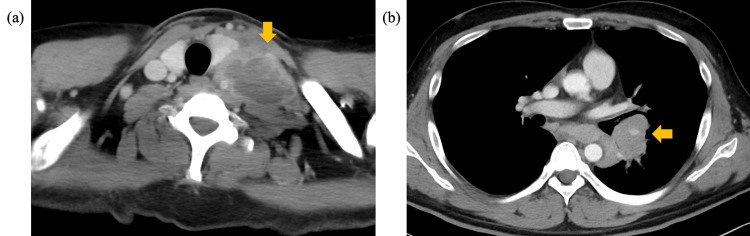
Computed tomography (CT) of the left cervical lymph node and the left hilar lymph node. (a) Contrast-enhanced CT on day 112; the left supraclavicular lymph node was enlarged (yellow arrow). (b) Contrast-enhanced CT on day 176; enlarged left hilar lymph nodes were identified (yellow arrow).
